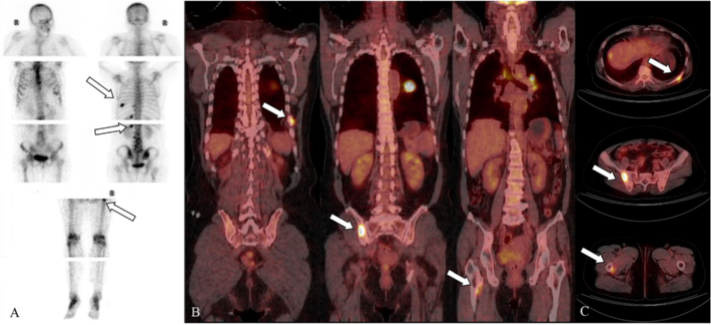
A 54-year-old woman with left lung cancer. Bone scanning (BS) shows focally increased uptake in the left ninth, tenth, and twelfth posterior ribs, three to five lumbar vertebrae and nearby regions of the lesser trochanter of the right femur (A). PET-CT revealed increased 18FDG intake at the left tenth posterior rib, right ilium, and right femur; the maximum standardized uptake value was 12.6 (B and C). More metastatic lesions were detected using 99mTc-MDP BS than 18F-FDG PET-CT.
